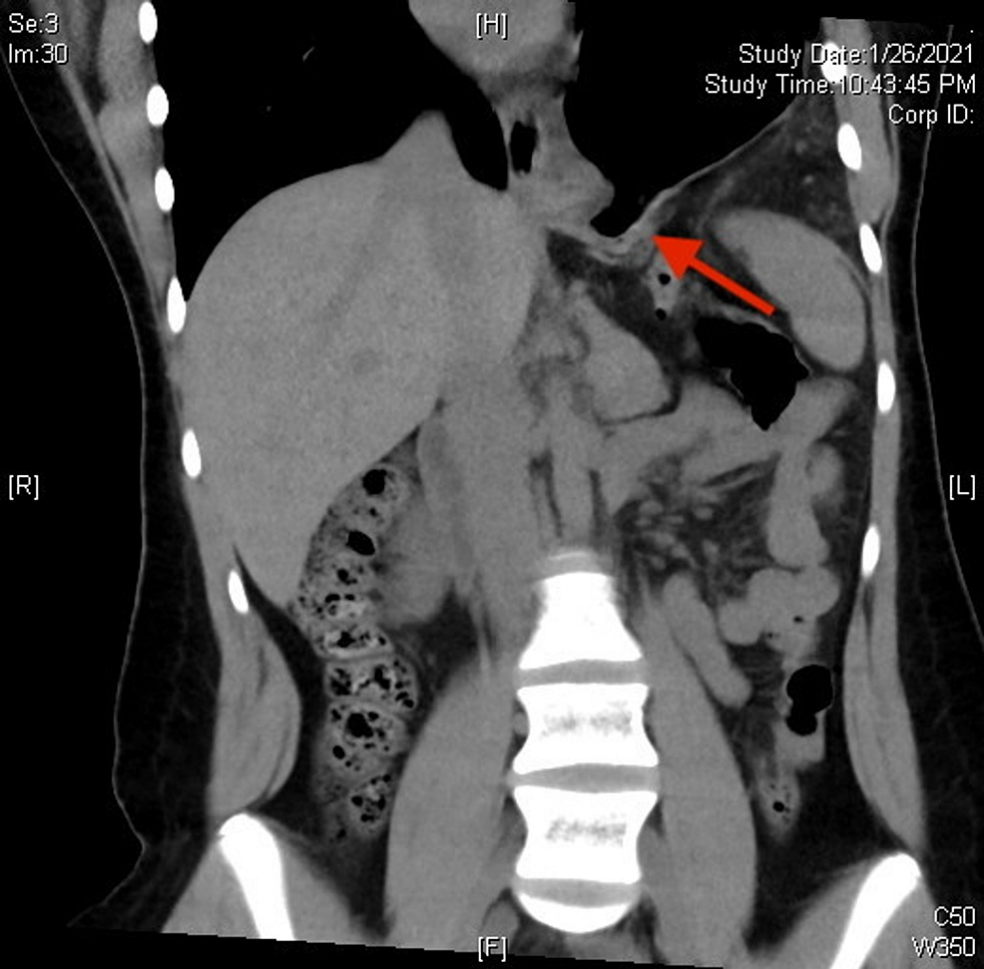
CT scan showing bowel passing through the diaphragmatic defect into thoracic cavity
